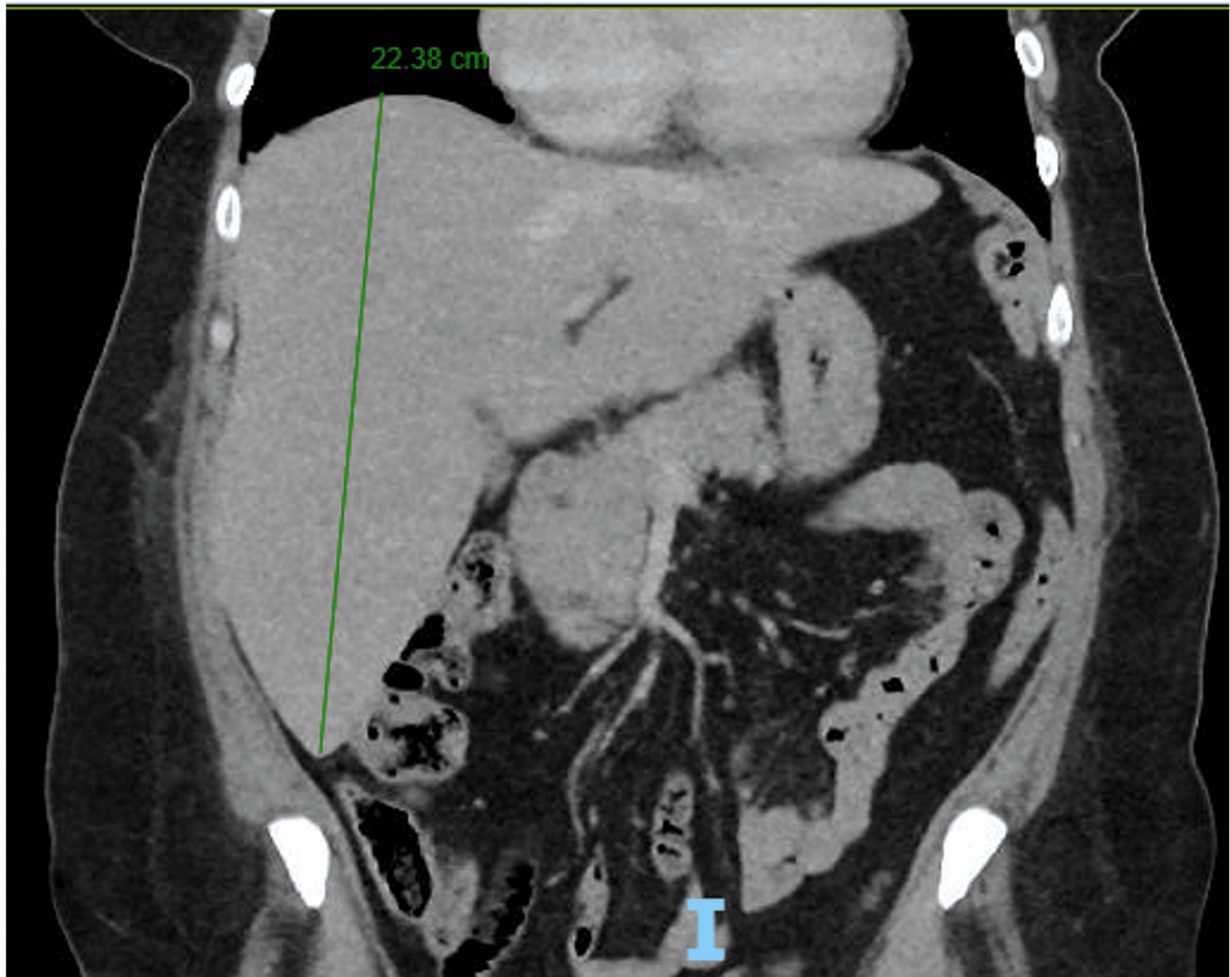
CT scan of the chest, abdomen, and pelvis for hepatomegaly Coronal view of the chest, abdomen, and pelvis. Liver measuring 22.38 cm (green line) I, Inferior
